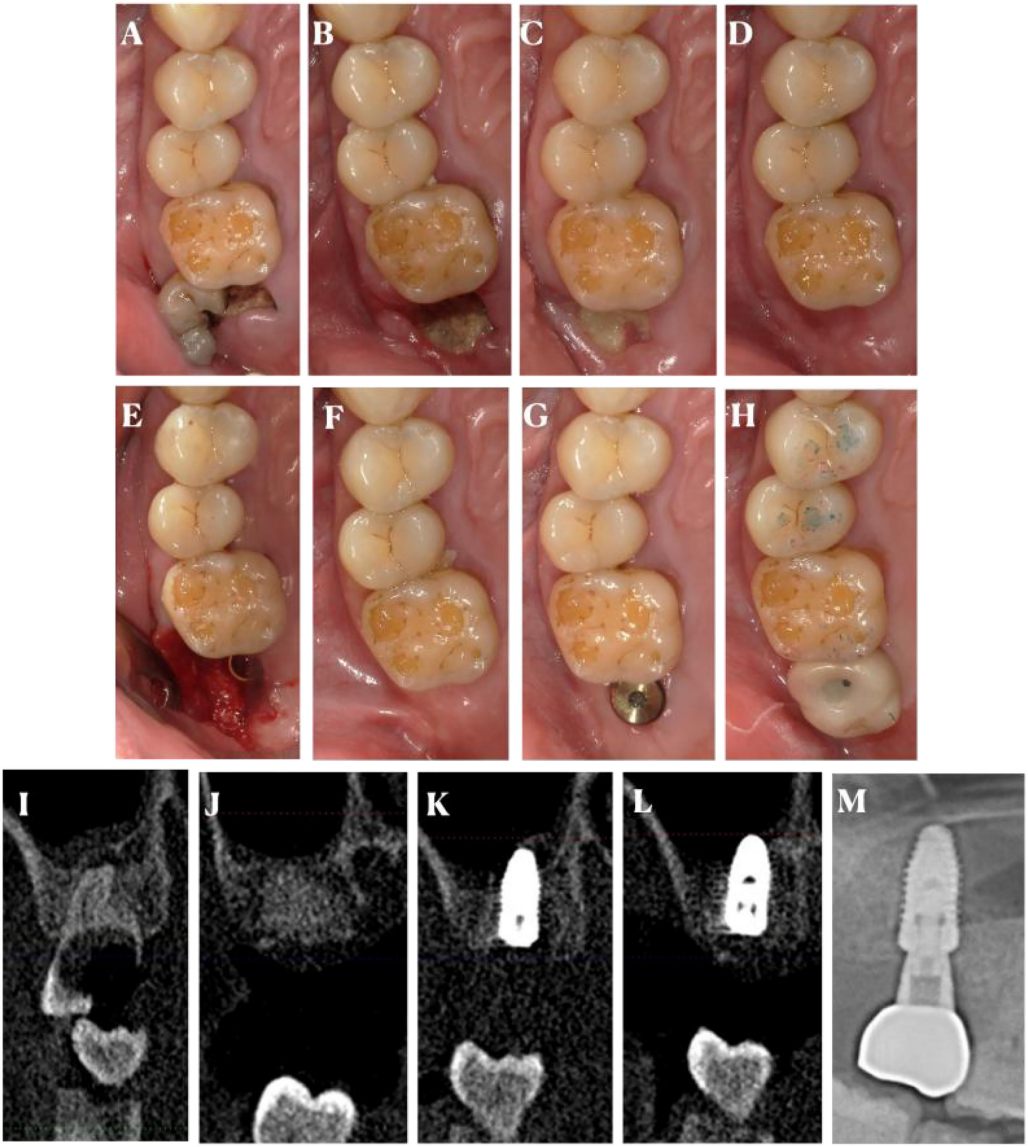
The procedures of the right side of #17. Intraoral photos (A), before tooth extraction; (B), after removed sutures; (C), 1-month follow-up; (D), before implant insertion; (E), after implant insertion; (F), before second stage operation; (G), after second stage operation; (H), after implant restoration; Radiograph (sagittal view of CBCT scan) (I), before tooth extraction; (J), before implant insertion; (K), after implant insertion; (L), before second stage operation; (M), after implant restoration.
